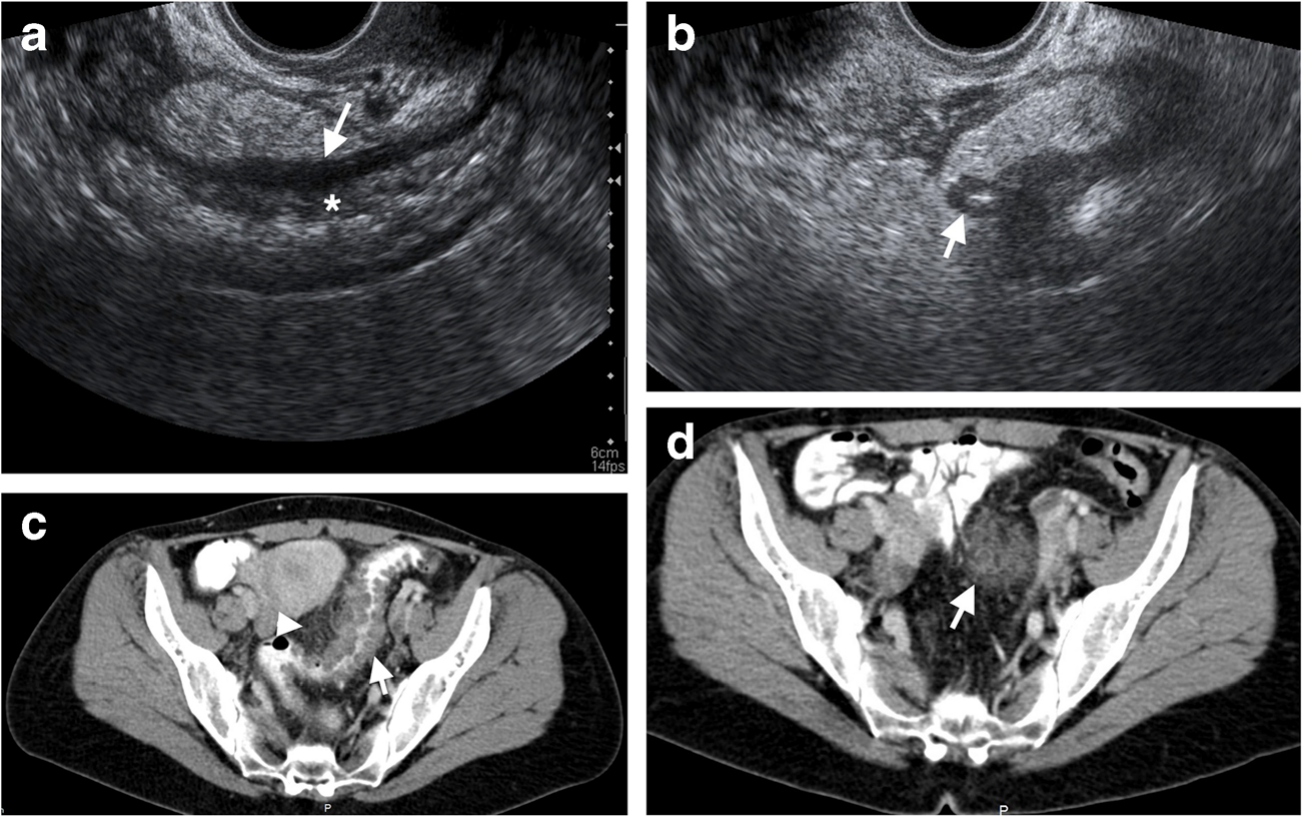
Phlegmon in a 38-year-old female with diverticulitis. a Endovaginal US reveals diffuse thickening and edema of the rectum and sigmoid colon mucosa (*) and muscular wall (*arrow*). b A diverticulum (*arrow*) with wall thickening, hyperechoic content (fecalith) and surrounding edematous fat tissue represents diverticulitis. c Axial contrast-enhanced CT of the same patient demonstrates multiple diverticulum with wall edema in distal sigmoid colon (*arrow*) and pericolonic fat stranding (*arrowheads*) representing diverticulitis. d Axial contrast-enhanced CT image at the superior level reveals a phlegmon with a hyperattenuating appearance (*arrow*) compared to adjacent pelvic fat
